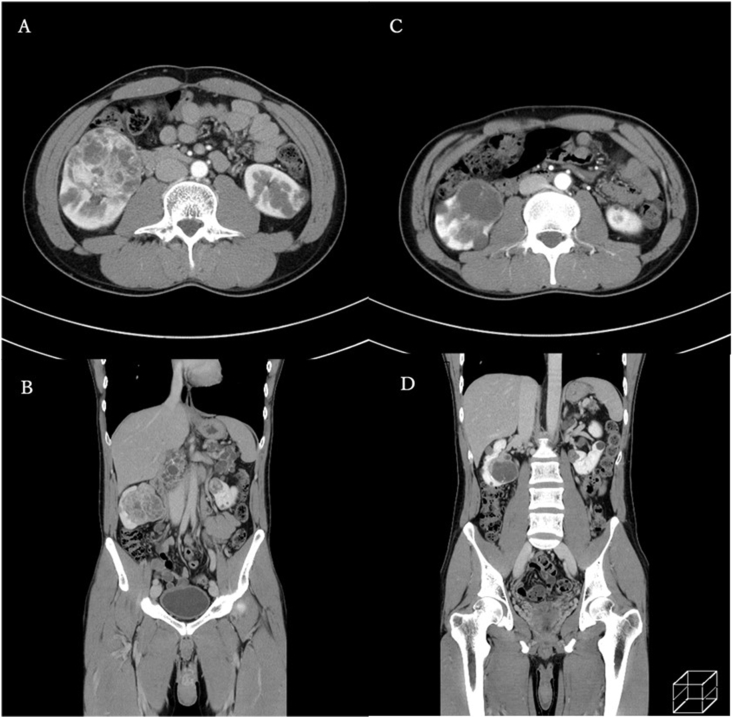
Dynamic computed tomography revealing multiple bilateral renal tumors with increased vascularity (largest diameter, 60 mm) before axitinib therapy (A: axial view, B: coronal view). Renal tumors exhibited a smaller size and diminished vascularity after 3 months of axitinib therapy (C: axial view, D: coronal view).
